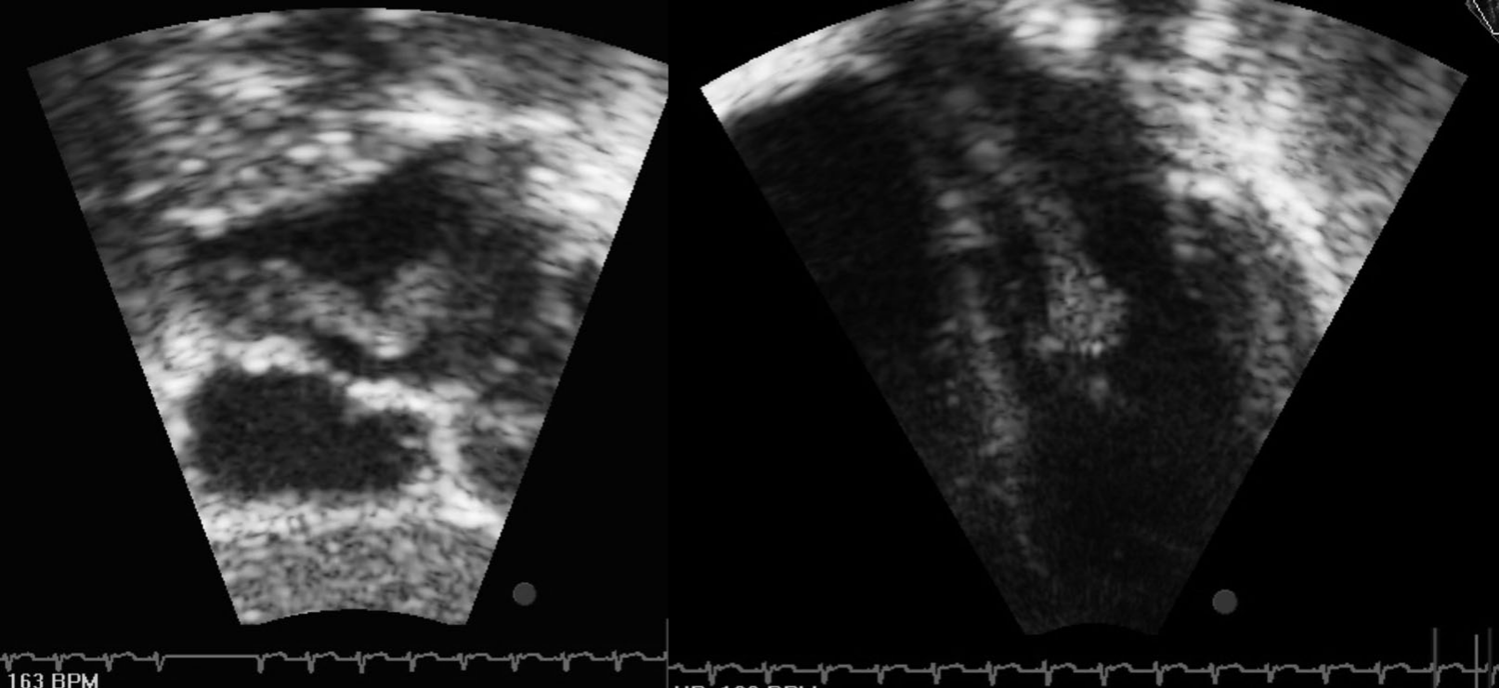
Trans-thoracic echocardiographic subcostal and parasternal views. A voluminous left atrial intracavitary pedunculated mass prolapsing during the diastole from the right lower pulmonary vein into the left ventricle through the mitral valve.
